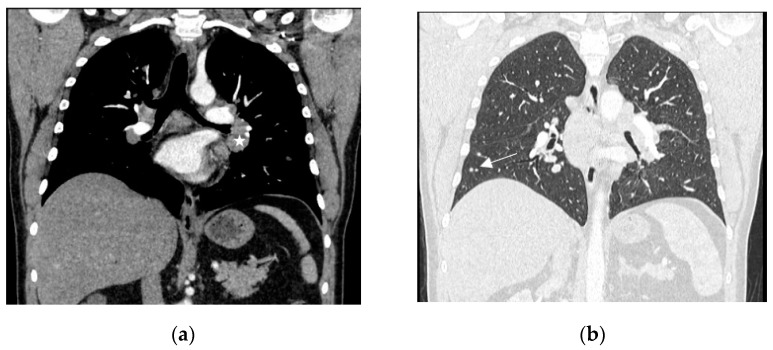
Computed tomography of the chest, with contrast enhancement, coronal plane, mediastinal window (a), lung window (b). Enlarged mediastinal and hilar lymph nodes ((a)—white asterisk). Small nodules in the right lower lobe ((b)—white arrow).
